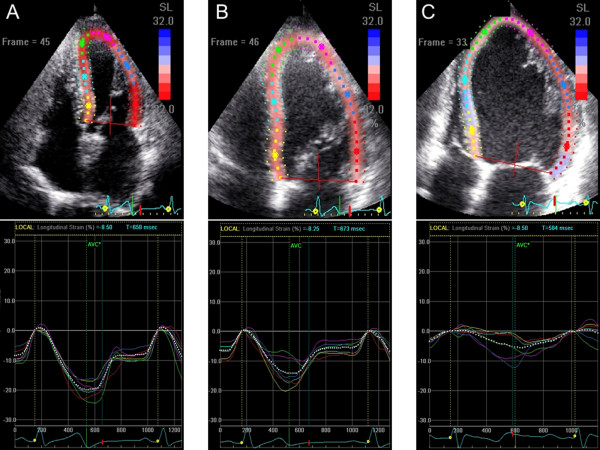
Examples of LV strain imaging in the apical four-chamber view (top) and corresponding time-strain curves (bottom) from a normal subject (A), a patient with OMI in the territory of left anterior descending artery and low plasma BNP level (B), and a patient with OMI in the all 3 coronary artery territories and high plasma BNP level (C).
